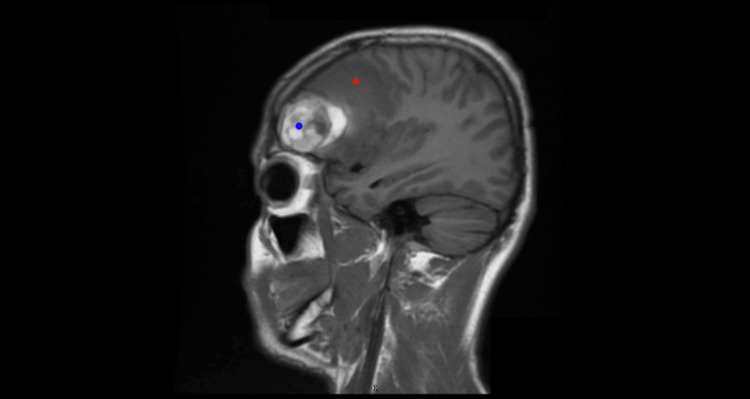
MRI (sagittal view) showing right frontal hemorrhage (blue circle) with edema (red triangle).
